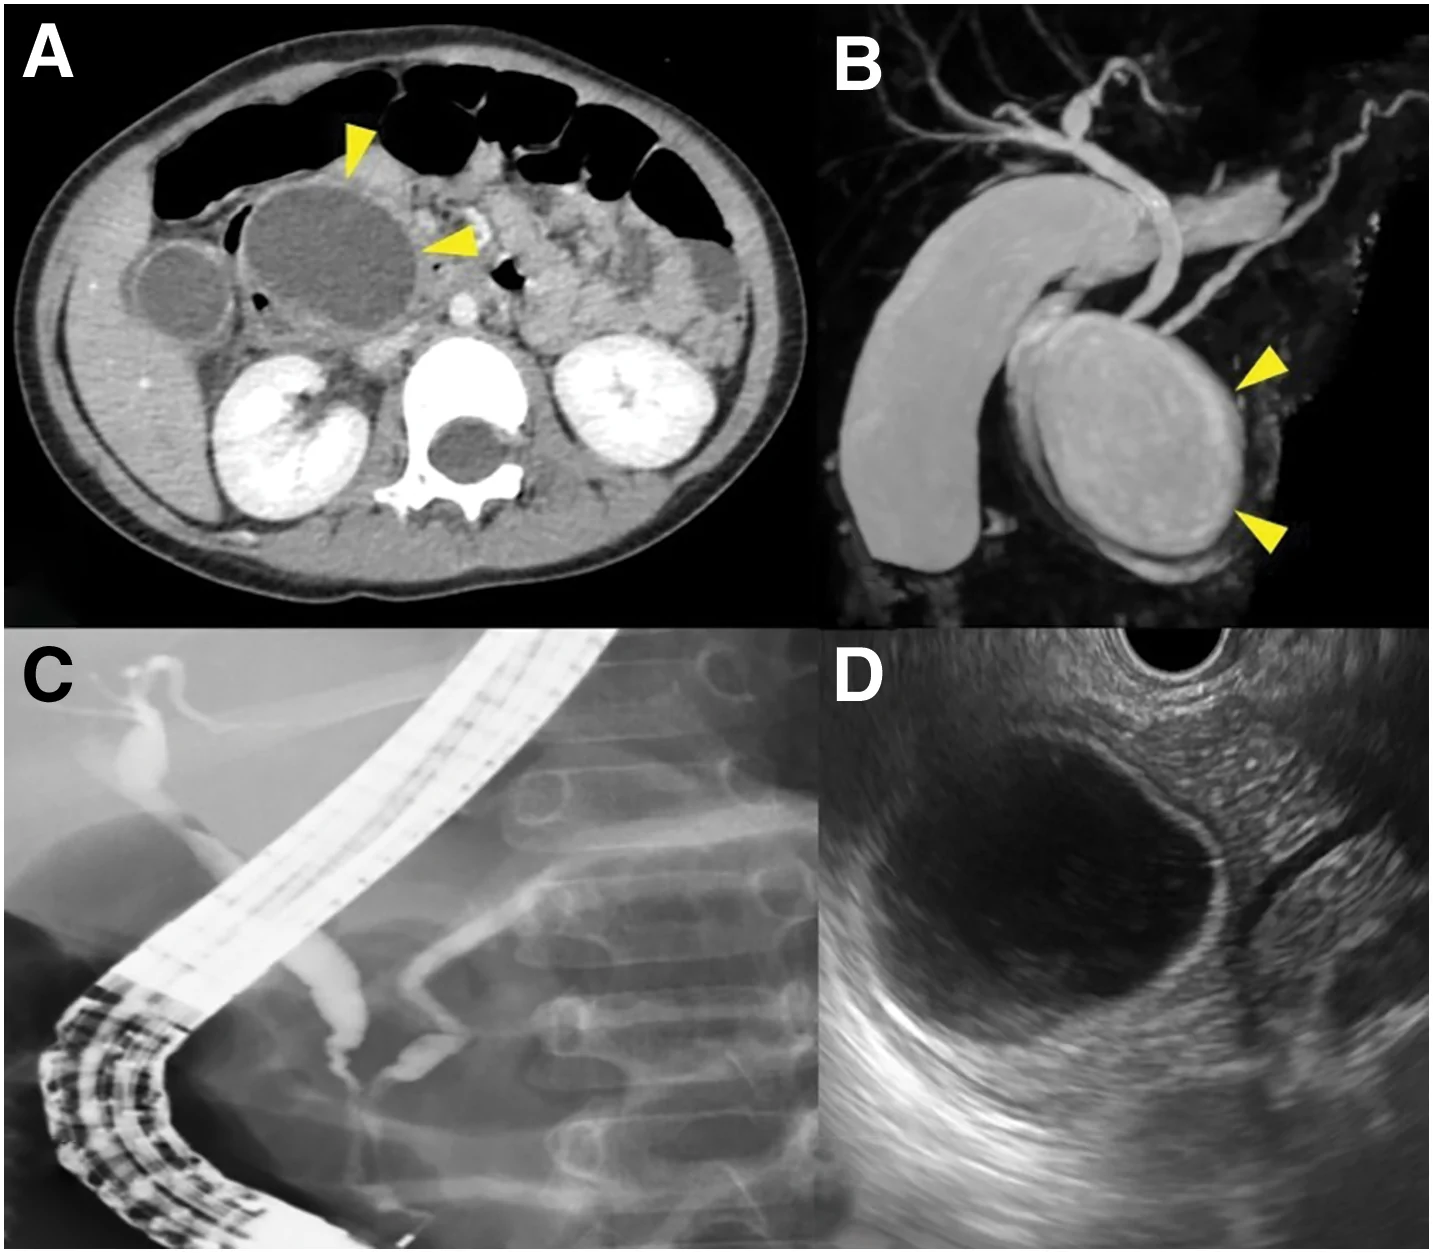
Preoperative imaging. (A, B) CT and MRCP: A cystic lesion was observed in the region of the pancreatic head (arrowheads). (C) ERCP: The cystic lesion did not communicate with the main pancreatic or bile ducts. No evidence of a pancreaticobiliary maljunction was observed. (D) EUS: The cyst wall exhibited layered structures with differing echogenicities, and mucinous contents were observed within the lesion. Continuity within the pancreas was not clearly identified, suggesting that the lesion likely originated outside the pancreas. ERCP, endoscopic retrograde cholangiopancreatography; EUS, endoscopic ultrasonography; MRCP, magnetic resonance cholangiopancreatography
